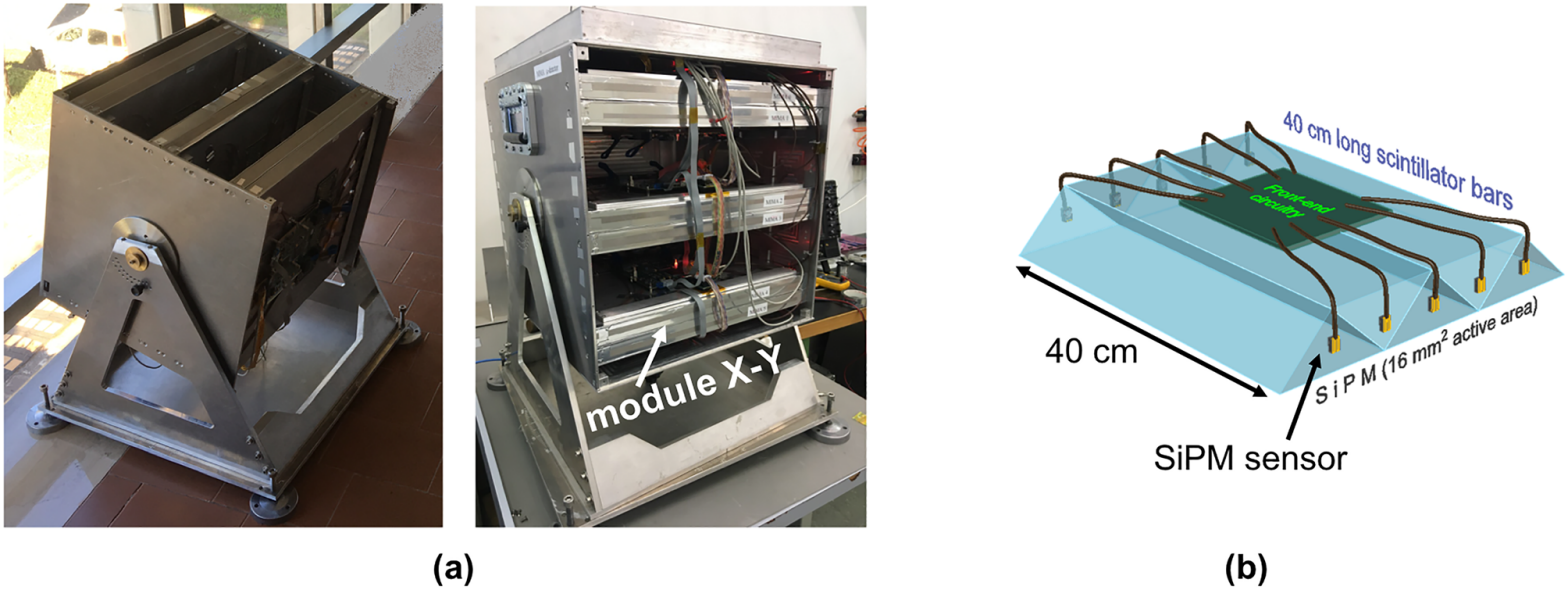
The MIMA muon tracker employed for the muon radiography measurements in the Temperino mine. In (a) the detector with the structure of 3 $$X-Y$$ module of tracking planes. In (b) the structure of a single plane consisting of triangular scintillator bars (for simplicity only five bars) is shown^1^.
